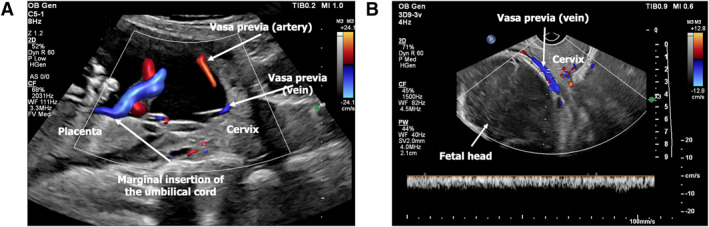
(A) Transabdominal two‐dimensional ultrasonography confirming type II vasa previa: Posterior placenta with marginal insertion of the umbilical cord and two previa vessels (one artery and one vein). (B) Transvaginal ultrasonography confirming the vasa previa.
